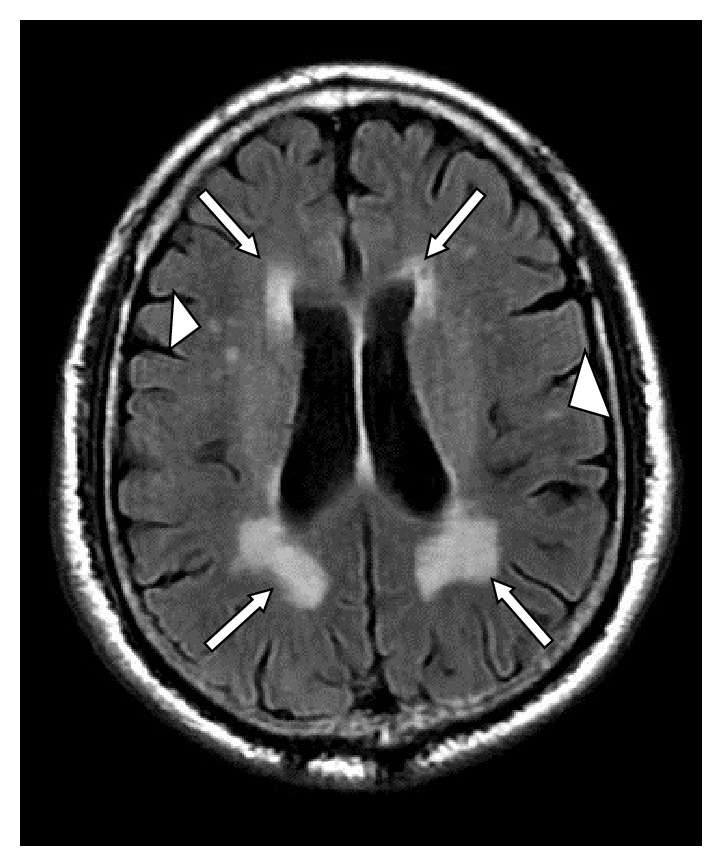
A 68-year-old male patient with type 2 diabetes mellitus for 20 years with abnormal periventricular white matter hyperintensities (WMHs) (arrows) and deep WMHs (arrowheads) on a FLAIR sequence and aortic arch PWV of 7.44 m/s and brachial artery FMD of 5.88%.
